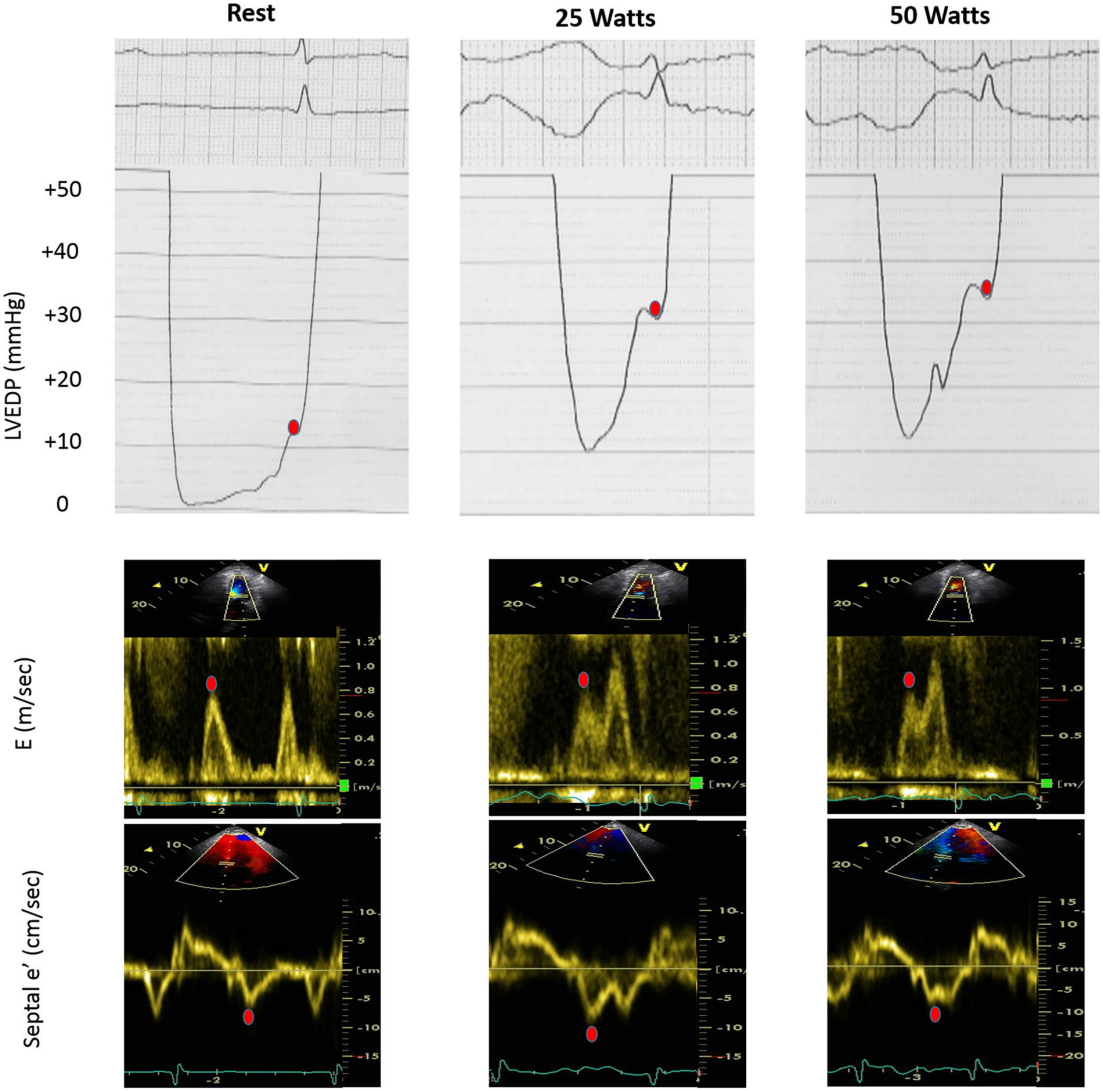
Example of Doppler and hemodynamic recordings for a patient included in this study with parameters at rest, 25 Watts and 50 Watts.
